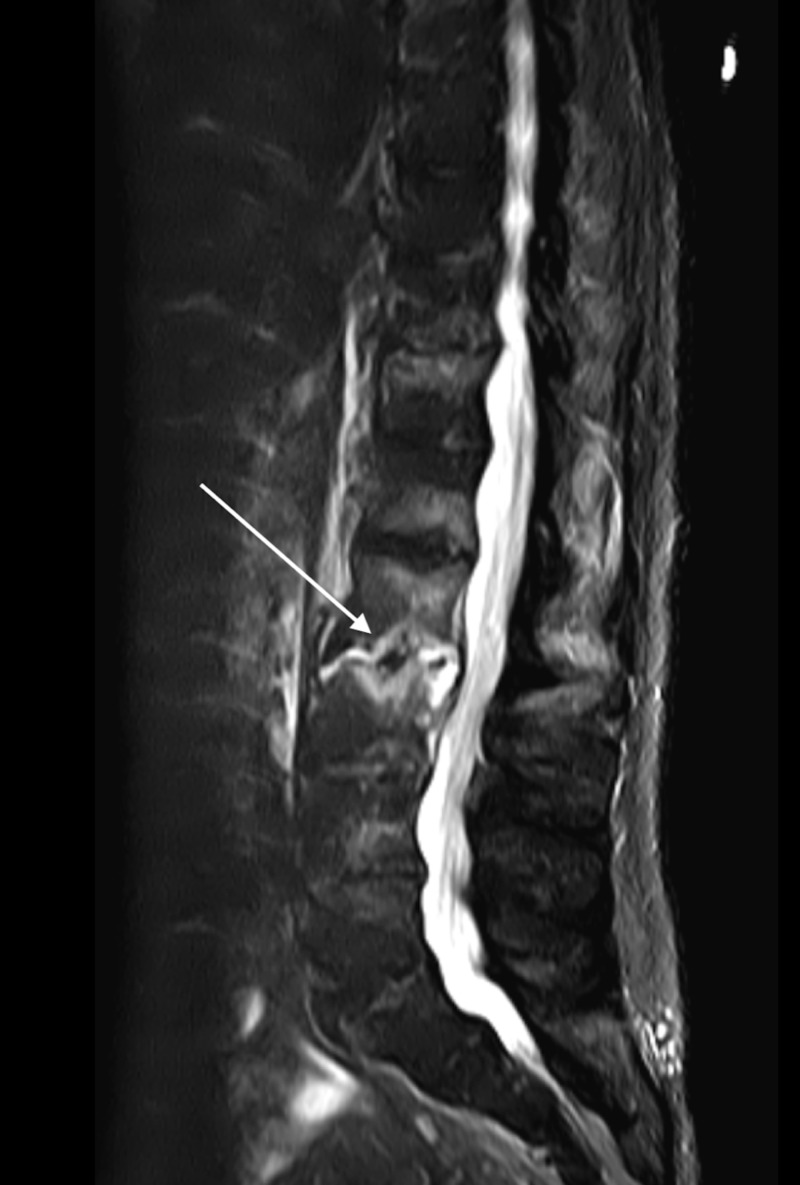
Magnetic Resonance Imaging with Contrast of the Lumbar Spine Sagittal image of the lumbar spine demonstrating acute discitis osteomyelitis with epidural phlegmon
